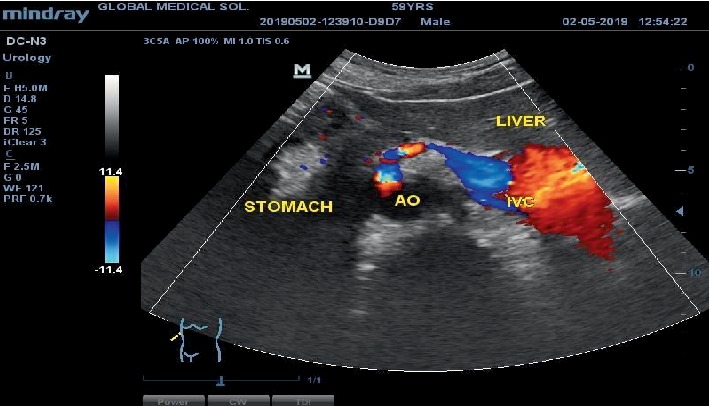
Colour Doppler ultrasound image of the epigastrium in transverse plane. This demonstrates a right-sided abdominal aorta (AO) and left sided inferior vena cava (IVC). Also showing a right-sided stomach and a left-sided liver, which is the reverse of their normal anatomical positions.
